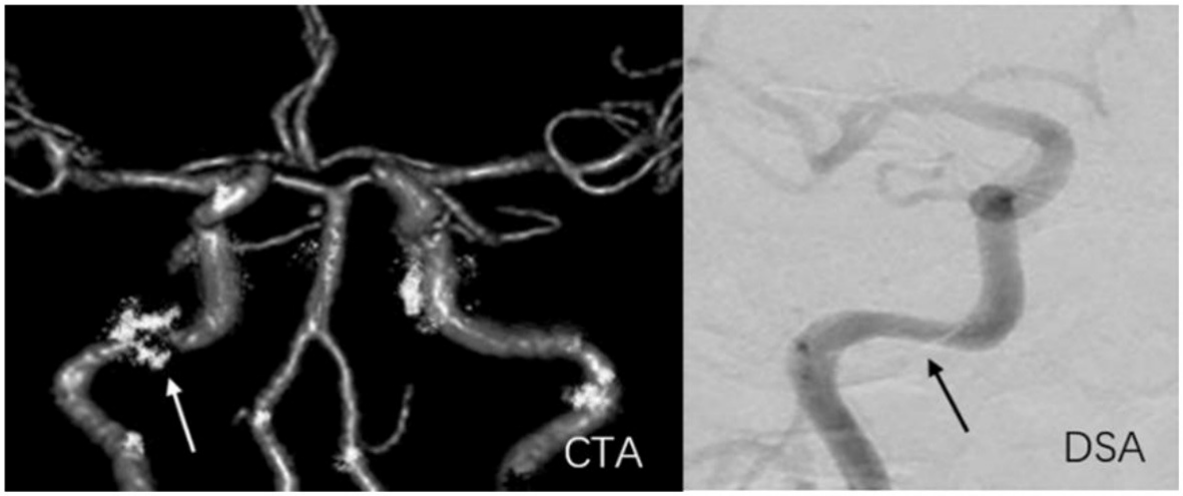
A 59-year-old man with stenosis and calcification on C2 of the right internal carotid artery. The arrow marks the location of the lesion. The degree of stenosis acquired on CTA (81.7%) exceeded that on DSA (50.3%).
